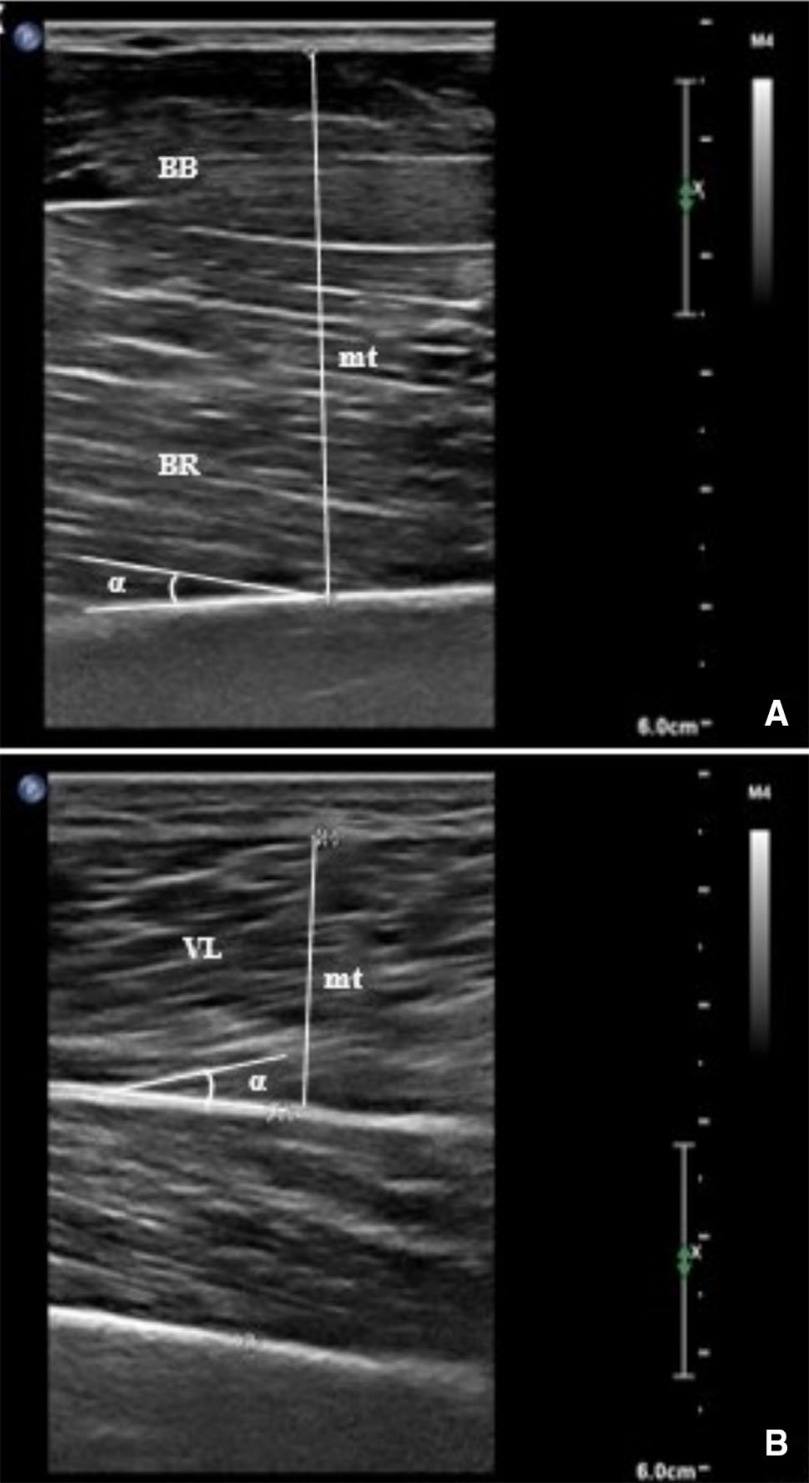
Sagittal ultrasound images: elbow flexors muscle thickness (mt) and pennation angle (*α*) of brachialis (a). Muscle thickness (mt) and pennation angle (*α*) of vastus lateralis (b)
